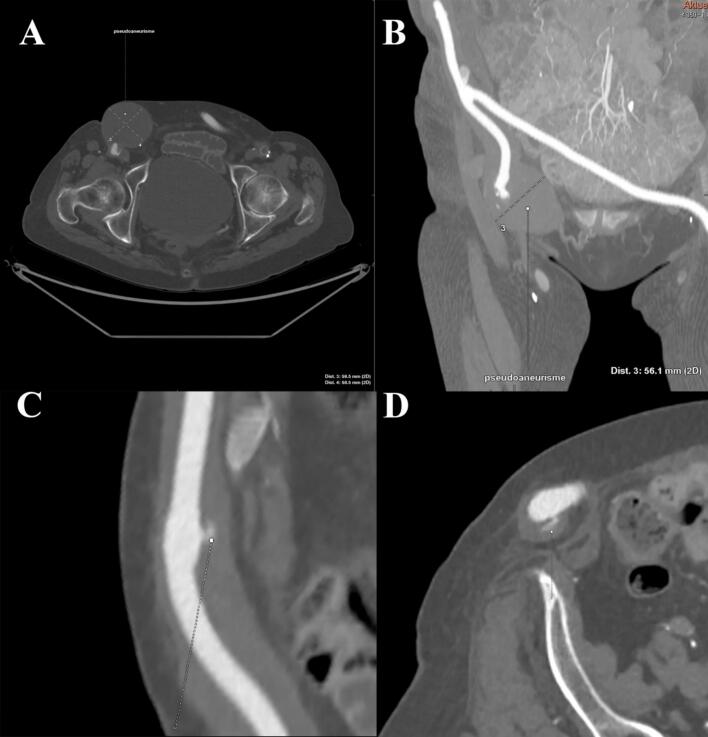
A and B: CT-angiography in axial and coronal plane, with Multiplanar Reconstruction (MPR) showing pseudoaneurysm forming in the anastomosis site between an axillofemoral bypass and fem-fem bypass. C and D: CT-angiography in arterial phase (axial plane), showing contrast extravasation from the defect of the posterior part of the prosthesis, causing the pseudoaneurysm.
